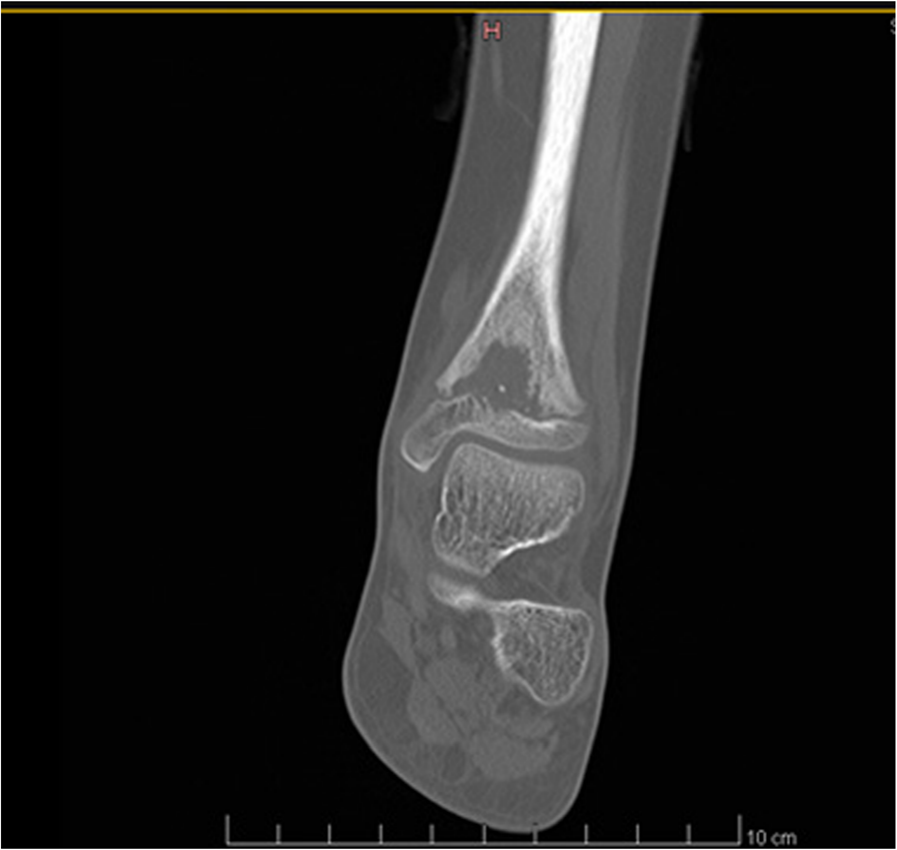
CT scan showing osteolytic lesion of the metaphysis of left tibia
